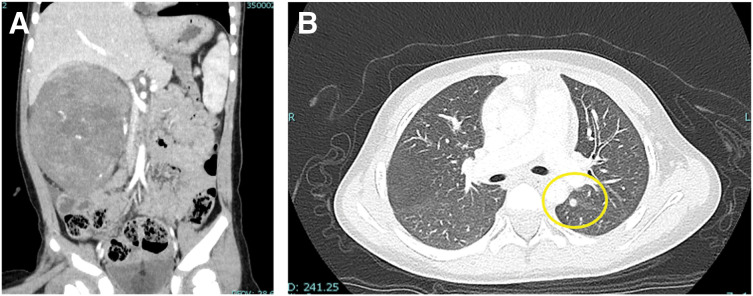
Initial contrast-enhanced CT findings at presentation. (A) Abdominal CT showing a 15-cm mass in the right kidney. (B) Chest CT demonstrating a 10-mm pulmonary metastasis in the left lung field (yellow circle).
